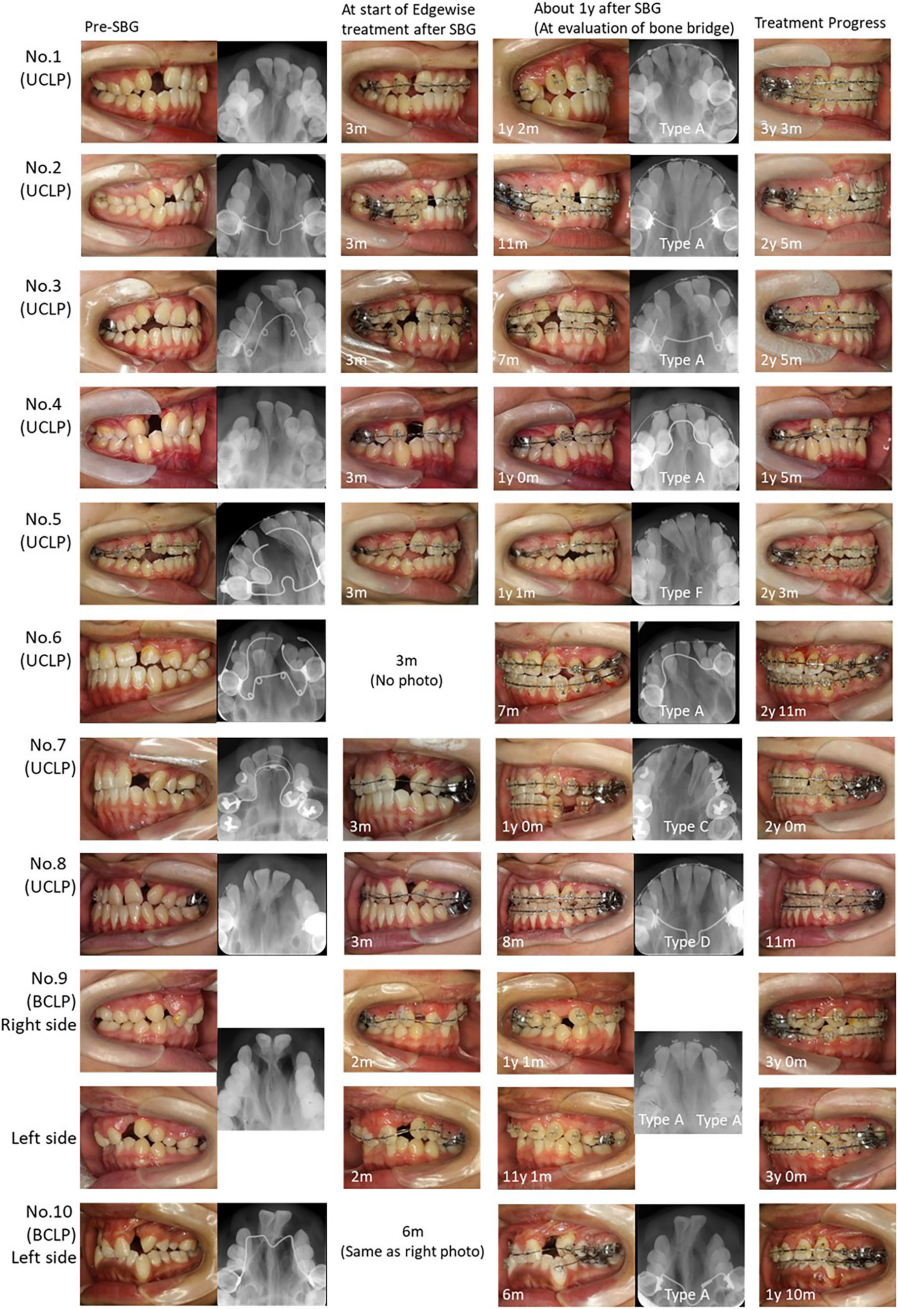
Treatment progress of all cases that underwent late‐stage SBG treatment (intraoral photographs of the cleft side and occlusal X‐rays). The figures indicate the number of years since SBG was implemented. The type of bone graft as seen on the occlusal radiograph is indicated per the Chelsea scale assessment. SBG, secondary bone grafting.
